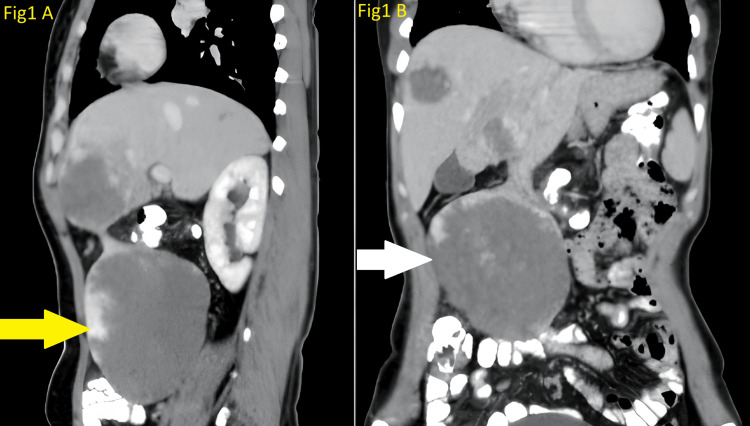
The CECT scan of the patient A: Saggital section view of CECT scan of the patient and a yellow arrow indicating hemangioma; B: Coronal section view of CECT scan of the patient and a white arrow indicating hemangioma CECT, contrast-enhanced computed tomography
